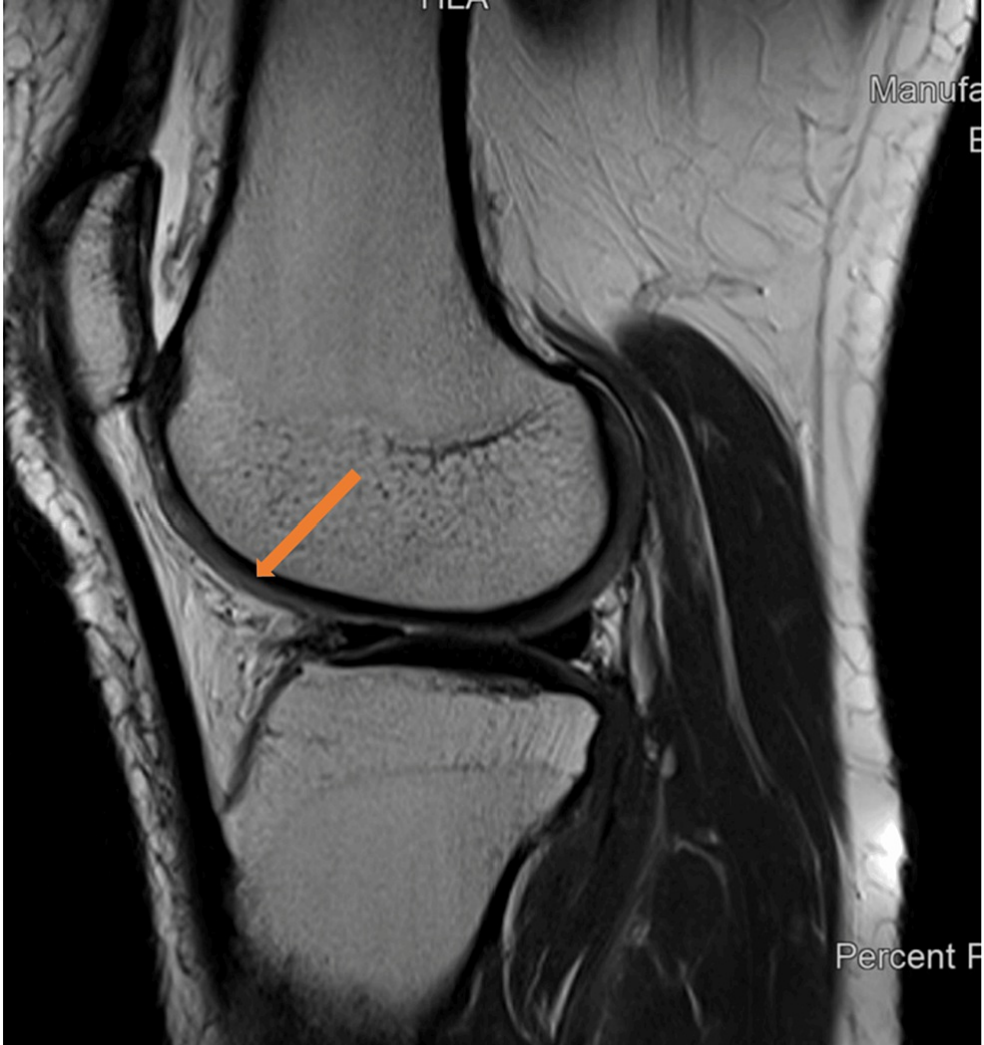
MRI sagittal view of knee joint showing degenerative changes and chondromalacia patella
